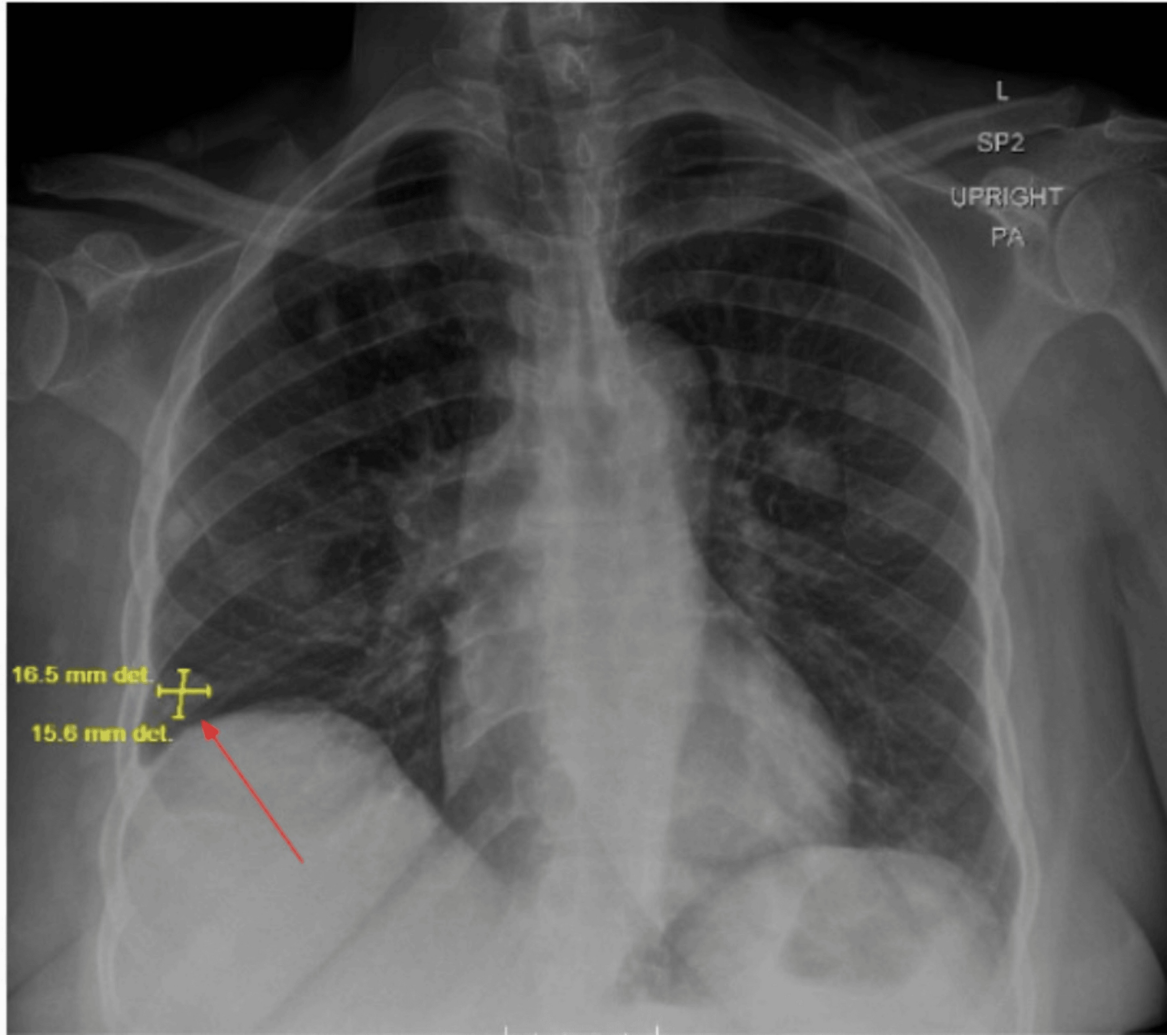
Chest radiograph showing multiple mass-like densities in the right lung A representative nodular density in the right lower lobe measures approximately 1.7 × 1.6 cm (thin red arrow).
